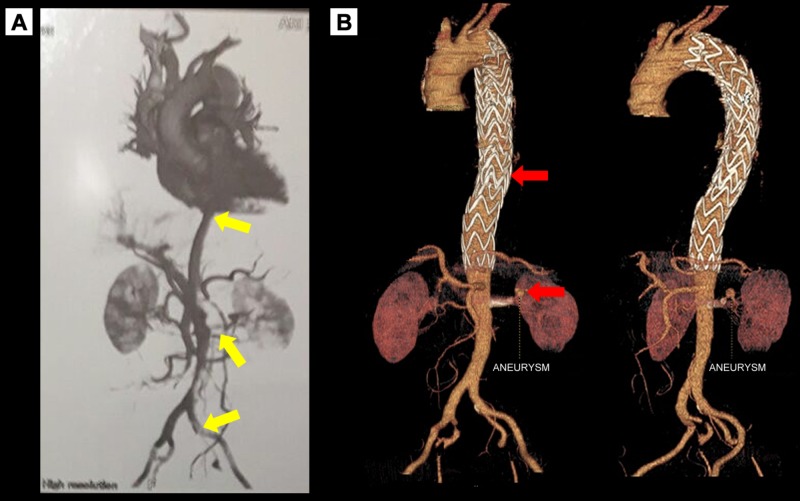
Preoperative CTA acute showing aortic dissection type B with exclusion of the left kidney, reduced lumen of the infrarenal aorta and iliac arteries, with discreet contrast filling of the left iliac artery indicated by yellow arrows (A) and three-dimensional CTA reconstruction showing postoperative endoprosthesis placement and a left renal artery aneurysm marked by the red arrows (B). CTA: computed tomography angiography
